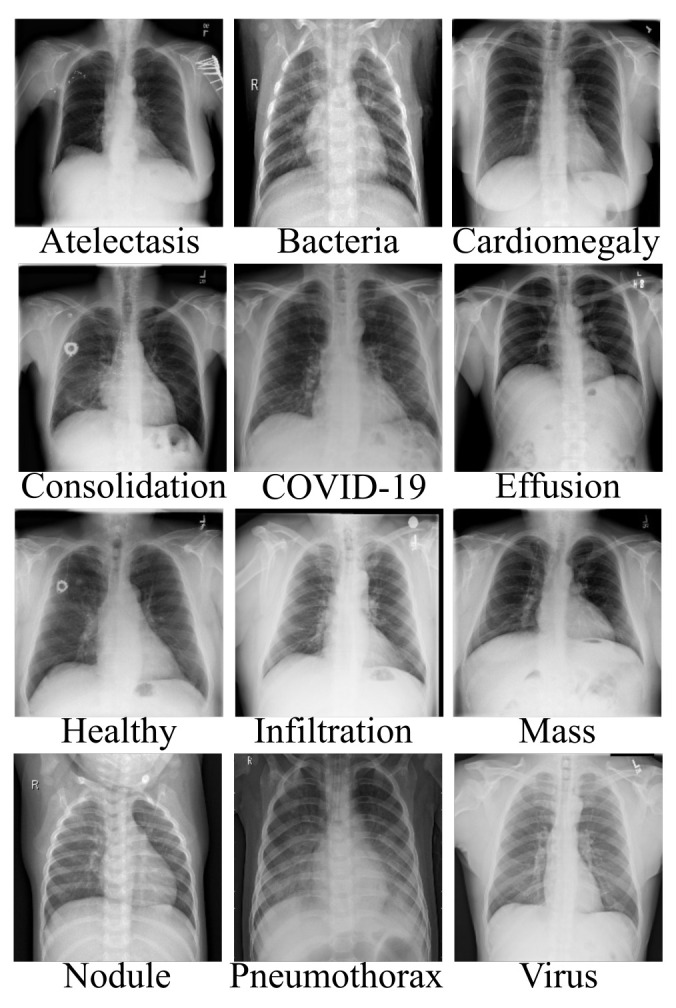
Data collection of chest X-ray images of different pneumonia-related illnesses including COVID-19.
